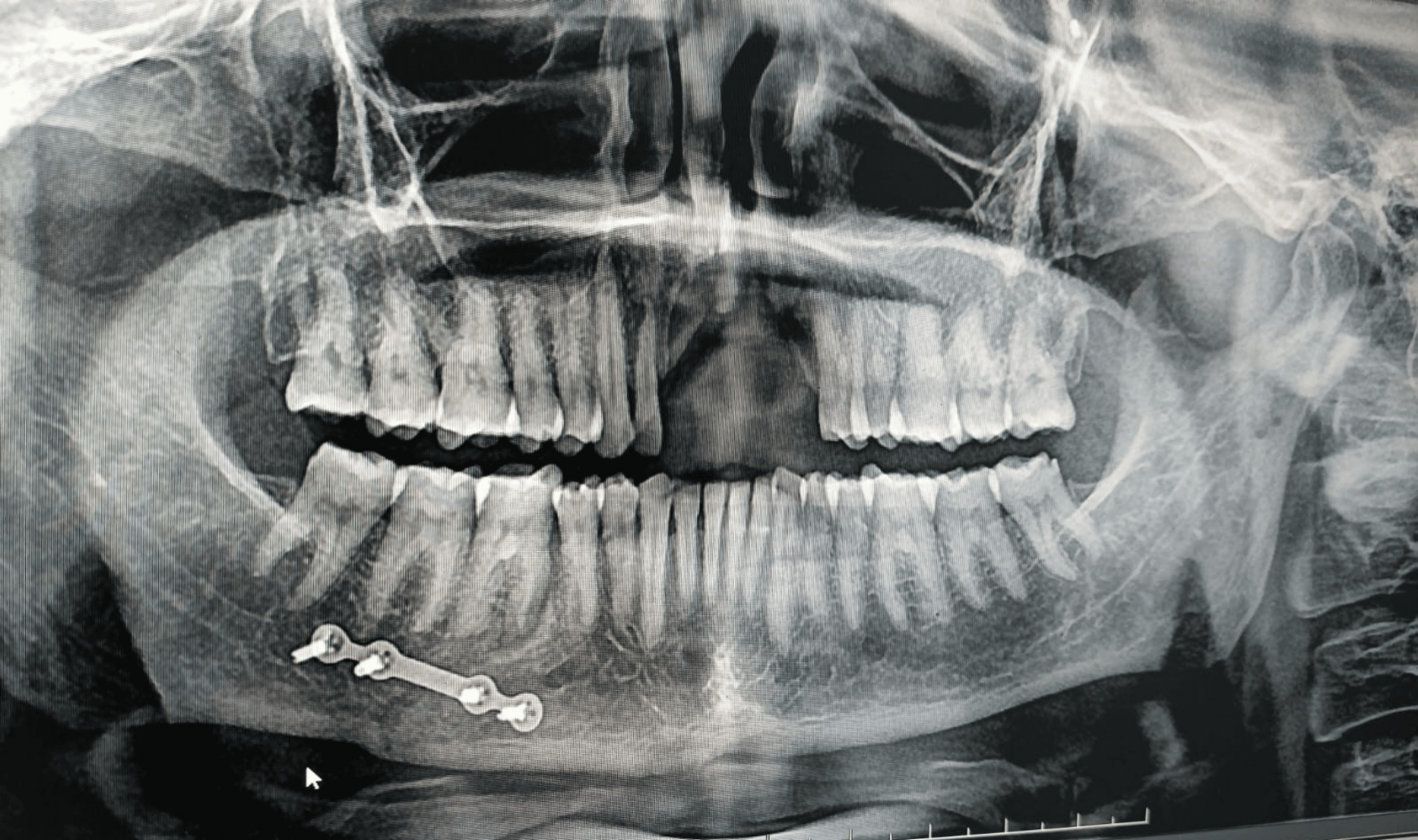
Panoramic radiograph (orthopantomogram) showing a well-aligned titanium fixation plate with four screws in the left mandibular body region.
